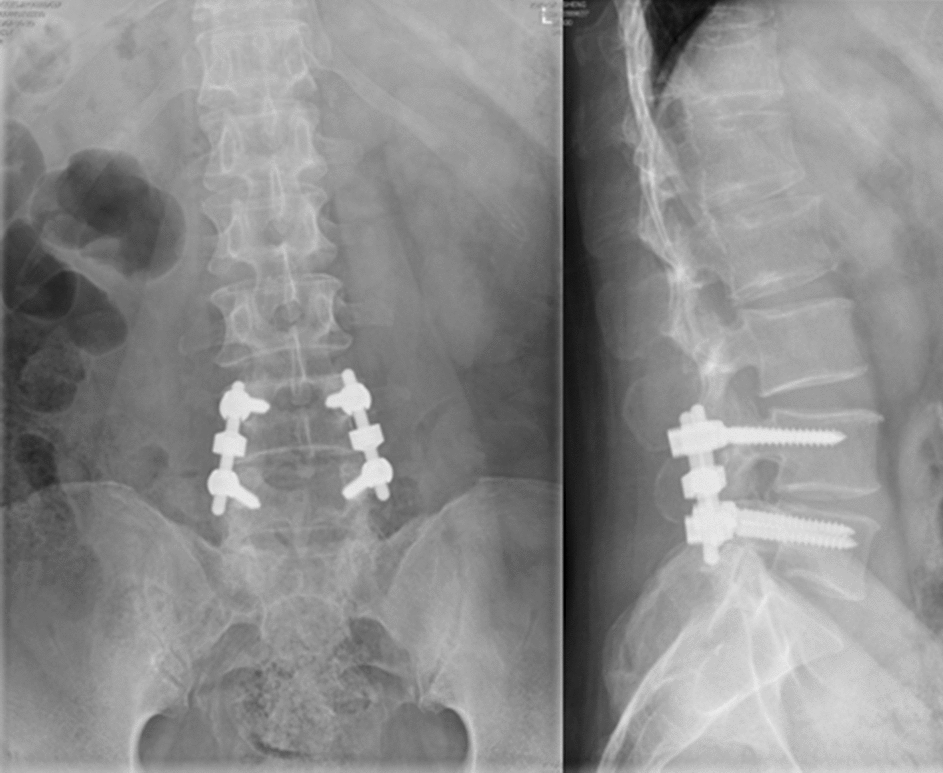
A 55-year-old male complained about his low back pain and intermittent claudication. Pre-operation X-ray indicated mild lumbar spondylolisthesis. He received decompression and semi-rigid non-fusion surgery
